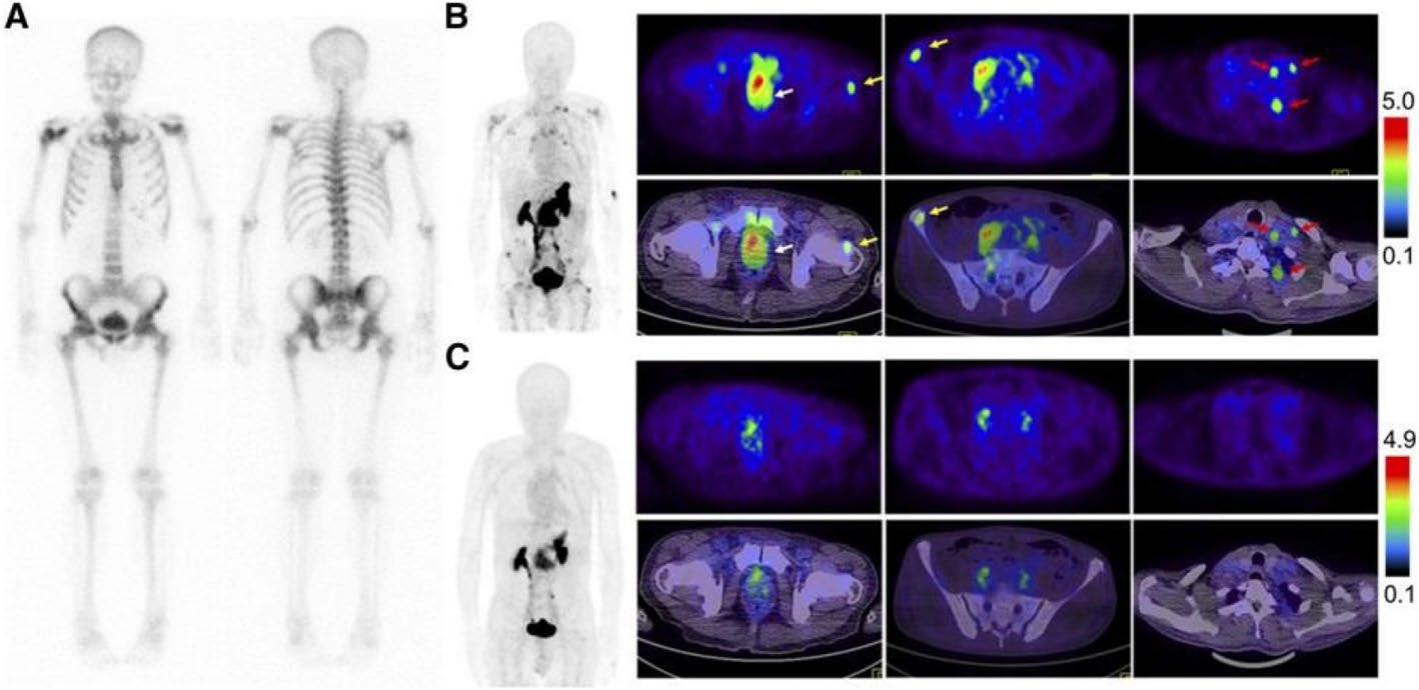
Comparison of ^99m^Tc-MDP bone scintigraphy (A), ^68^Ga-RM26 PET/CT (B), and ^68^Ga-BBN PET/CT (C) in a 73-y-old man diagnosed as having PC (white arrow) with lymph node involvement (red arrow) and bone metastasis (yellow arrow) before prostatectomy. ^68^Ga-RM26 PET/CT detected primary tumors, multiple lymph node involvement, and bone metastasis lesion, whereas those lesions did not significantly show up on ^99m^Tc-MDP bone scintigraphy and showed extremely mild uptake on ^68^ Ga-BBN PET/CT. *This*
